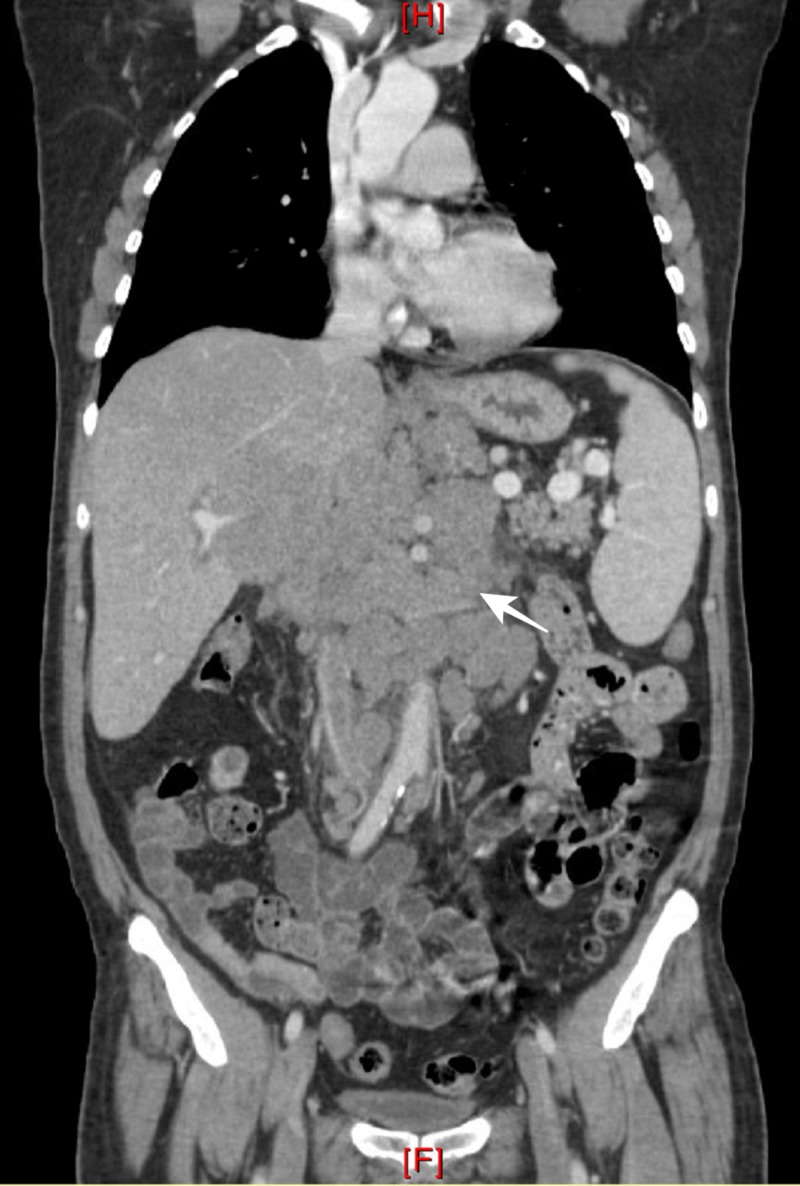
Computed tomography coronal view Extensive lymphadenopathy throughout the chest, abdomen, and pelvis with a large conglomerate
